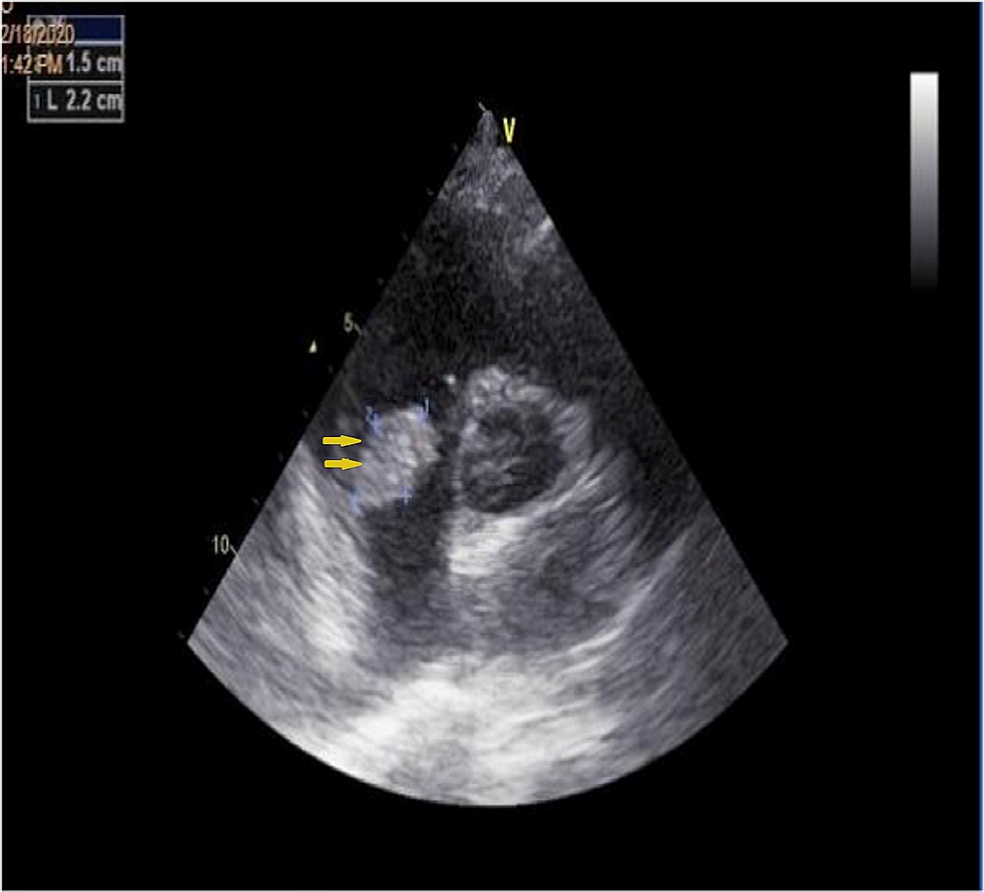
The short parasternal axis reveals bulky vegetation on the tricuspid valve.
